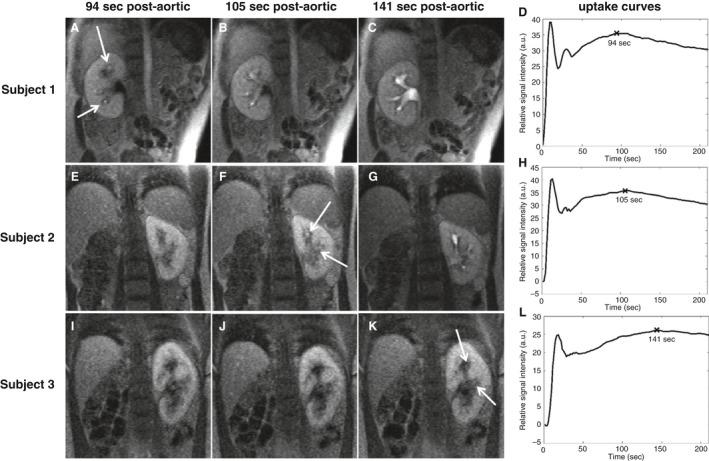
Dynamic contrast‐enhanced MR images and renal uptake curves in three study subjects. Examples shown represent a normal (Subject 1, uptake interval 94 sec), a slower (Subject 2, 105 sec), and very slow (Subject 3, 141 sec) uptake phase. Each row shows parenchymal images for the subject at time points 94 sec, 105 sec, and 141 sec postaortic, and the corresponding uptake curve. The white arrows point to the areas of early excretion of contrast agent from the renal parenchyma into the collecting ducts. The “X” mark on the uptake curves (D, H, L) represent the end‐of‐uptake points (i.e., the maximum parenchymal enhancement) corresponding to images A, F, K, respectively.
